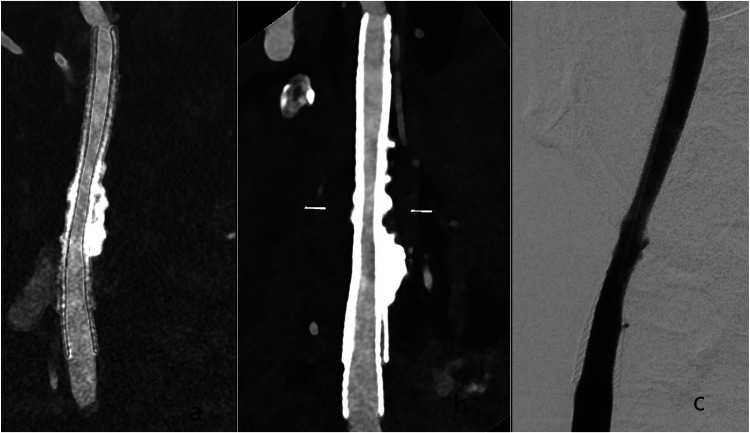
Postinterventional PCD-CTA with VMI and iodine reconstructions of an in-stent stenosis treated with a second carotid stent in a patient with calcified plaques at the carotid bifurcation. The patient has two carotid WallStents^®^ (double layer) in the right carotid artery. A is an iodine reconstruction with Bv56; B is an VMI with Bv56 at 40 keV with Bv56 of the right carotid artery; C is a p.a. 2D projections of the right carotid artery. Abbreviations: Bv: Body vascular kernel, keV: kiloelectron-volt, PCD-CTA: Photon-counting detector CT-Angiography, VMI: Virtual Monoenergetic Imaging.
